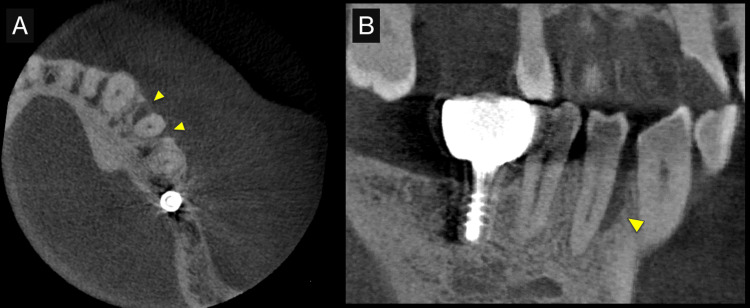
Preoperative cone-beam computed tomography (CBCT) images. (A) CBCT axial view, and (B) CBCT oblique sagittal view. CBCT images allowed the detection of the defect in 3D (arrowheads).
